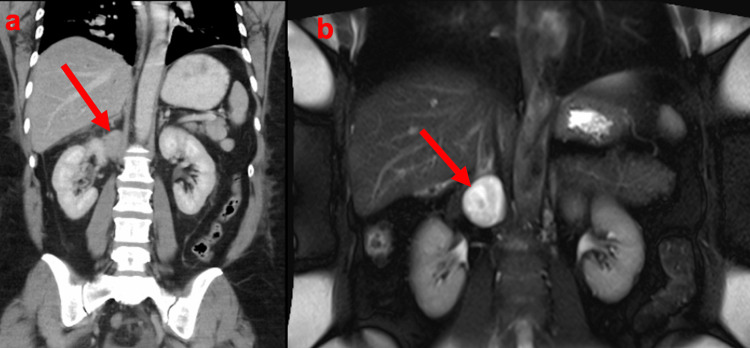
(a) Coronal CT and (b) MRI showing a 28 mm soft tissue mass (RBC – red arrow) adjacent to the right kidney CT: computed tomography; MRI: magnetic resonance imaging; RBC: retroperitoneal bronchogenic cyst
